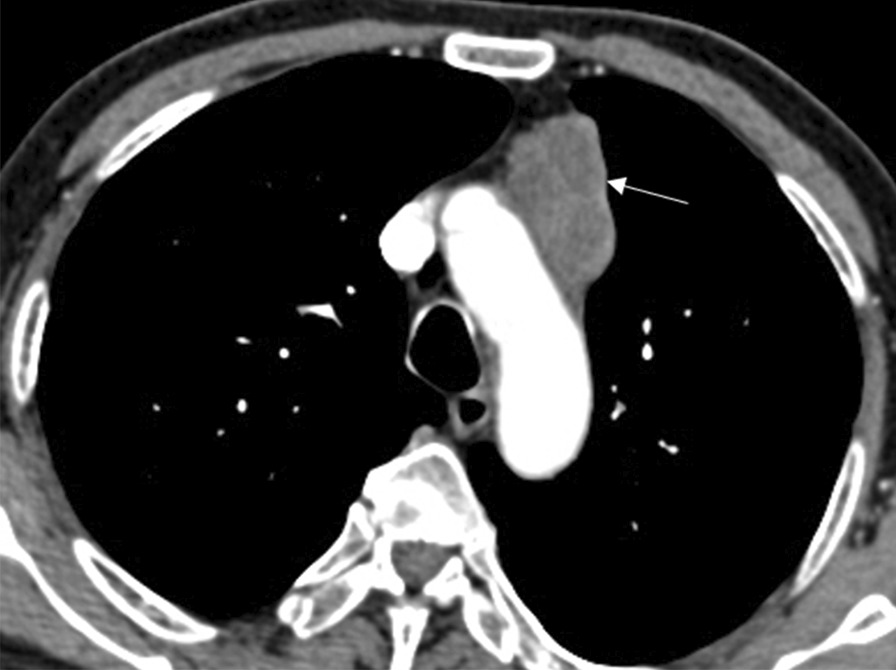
Chest CT. Chest CT shows an irregular contours mass in anterior mediastinum with mild heterogenetic enhancement (white arrow)
